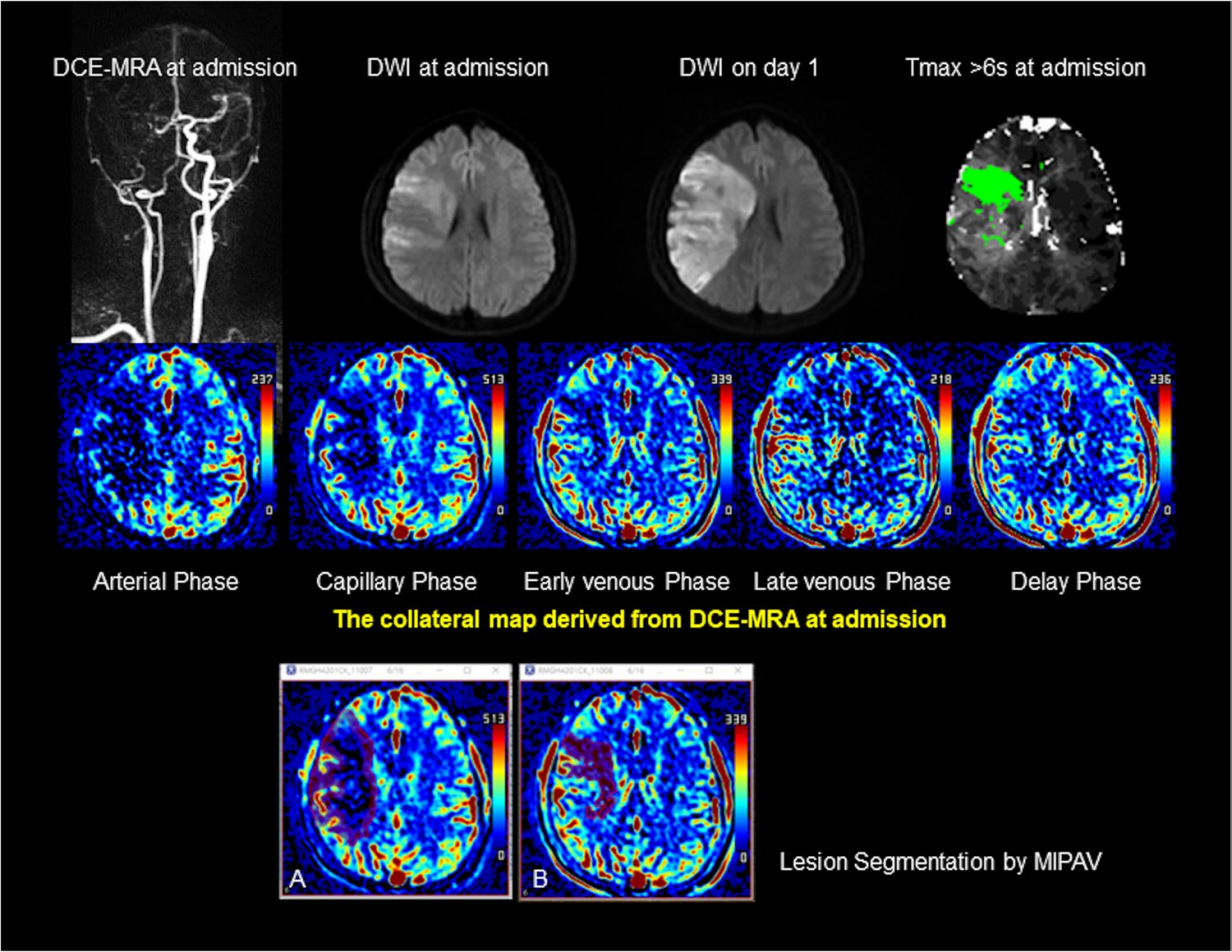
Case of intermediate collateral perfusion grade. Images of a middle-aged patient with occlusion of the right internal carotid and middle cerebral arteries demonstrated on dynamic contrast-enhanced MR angiography (DCE-MRA). The premorbid modified Rankin scale score of this patient was 0, and the National Institutes of Health Stroke Scale score at admission was 11. The patient underwent intravenous thrombolysis followed by intraarterial thrombectomy, but recanalization of the occluded arteries was not achieved. Diffusion-weighted imaging (DWI) at admission showed an acute infarction in the right middle cerebral artery territory. The collateral map derived from DCE-MRA at admission shows an intermediate collateral perfusion status (MR acute ischemic stroke collateral perfusion score of 2: collateral perfusion delay more than one-half of the middle cerebral artery territory in the capillary phase and equal to or less than one-half in the early venous phase). The DWI lesion extent at admission coincides with the hypoperfused lesion on the early venous phase of the collateral map, so the early venous phase is determined as the baseline lesion phase. DWI on day 1 shows that the lesion growth covers the entire hypoperfused lesion seen on the capillary phase of the collateral map at admission, so the capillary phase is determined as the phase_FU. A and B are images displayed on Medical Image Processing, Analysis, and Visualization (MIPAV; version 7.1.1; National Institutes of Health), showing the hypoperfused lesion painted on images in the capillary and early venous phases, respectively, for measuring the volume of the hypoperfused lesion of the collateral map. The precise collateral ratio was calculated as the ratio of the hypoperfused lesion volume in the phase_FU to the hypoperfused lesion volume in the phase_baseline. The lesion (green area) with a threshold of  > 6 s of the contralateral time-to-maximum (Tmax > 6 s) on MR perfusion imaging is similar to the baseline DWI lesion. The collateral map shows the extents of baseline lesion and final infarction precisely, but Tmax  > 6 s underestimates the final infarct extent
